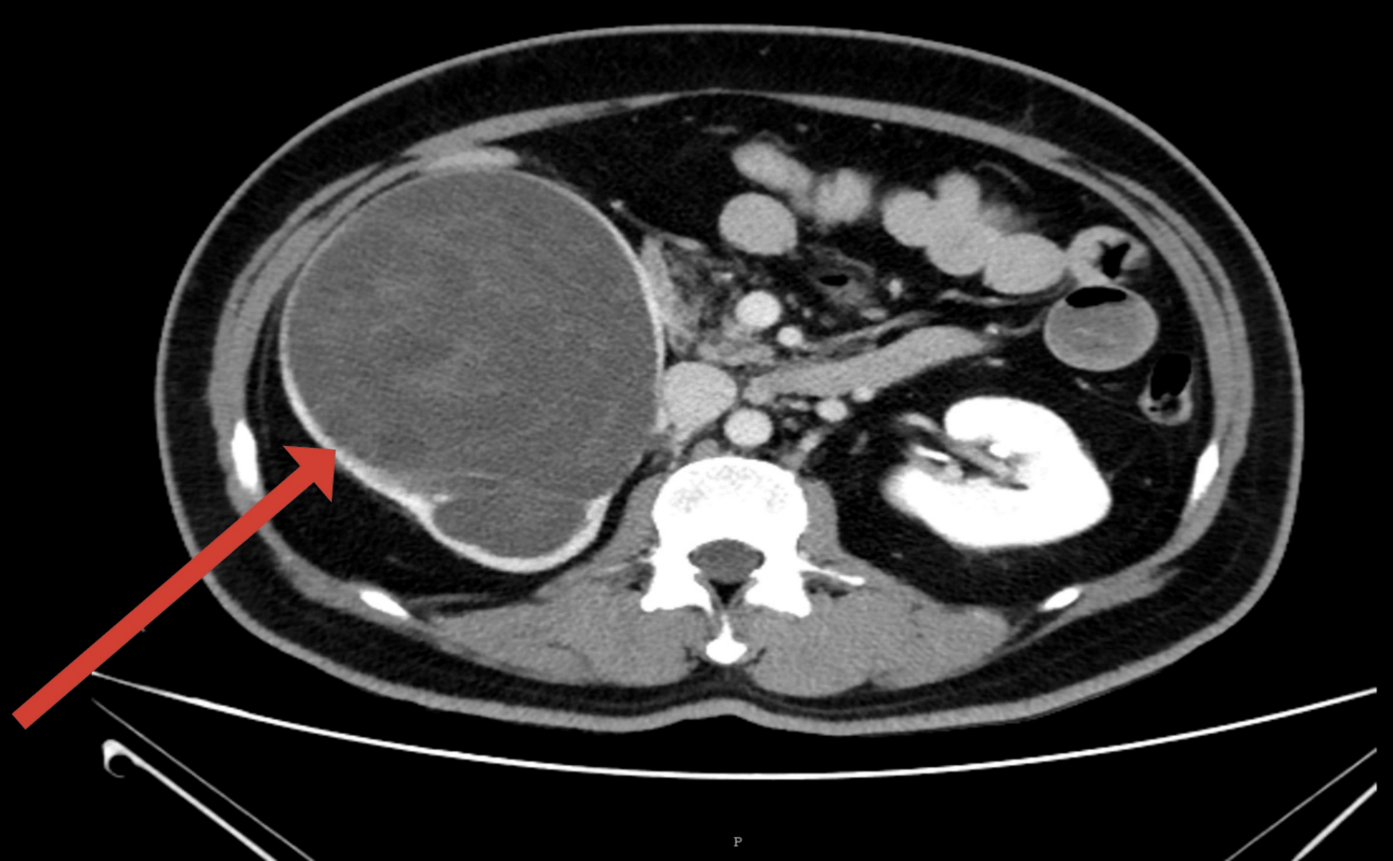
Axial contrast-enhanced CT image showing gross right hydroureteronephrosis with thinned-out renal parenchyma and no visible mass or septations. The arrow points to the right kidney, which is enlarged and appears cystic, without septations (radiologically mimicking hydronephrosis).
